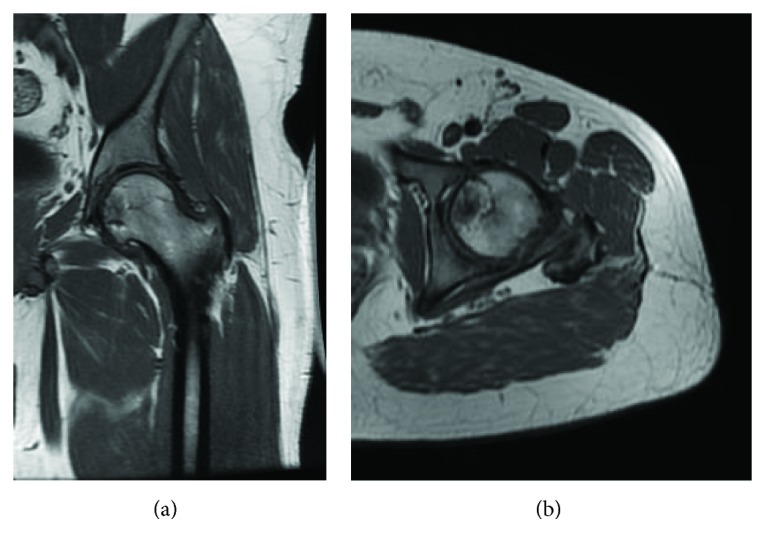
Postoperative MRI of the left hip at 6 years: (a) T1 AP view and (b) T1 axial view of a well-integrated osteochondral graft.
